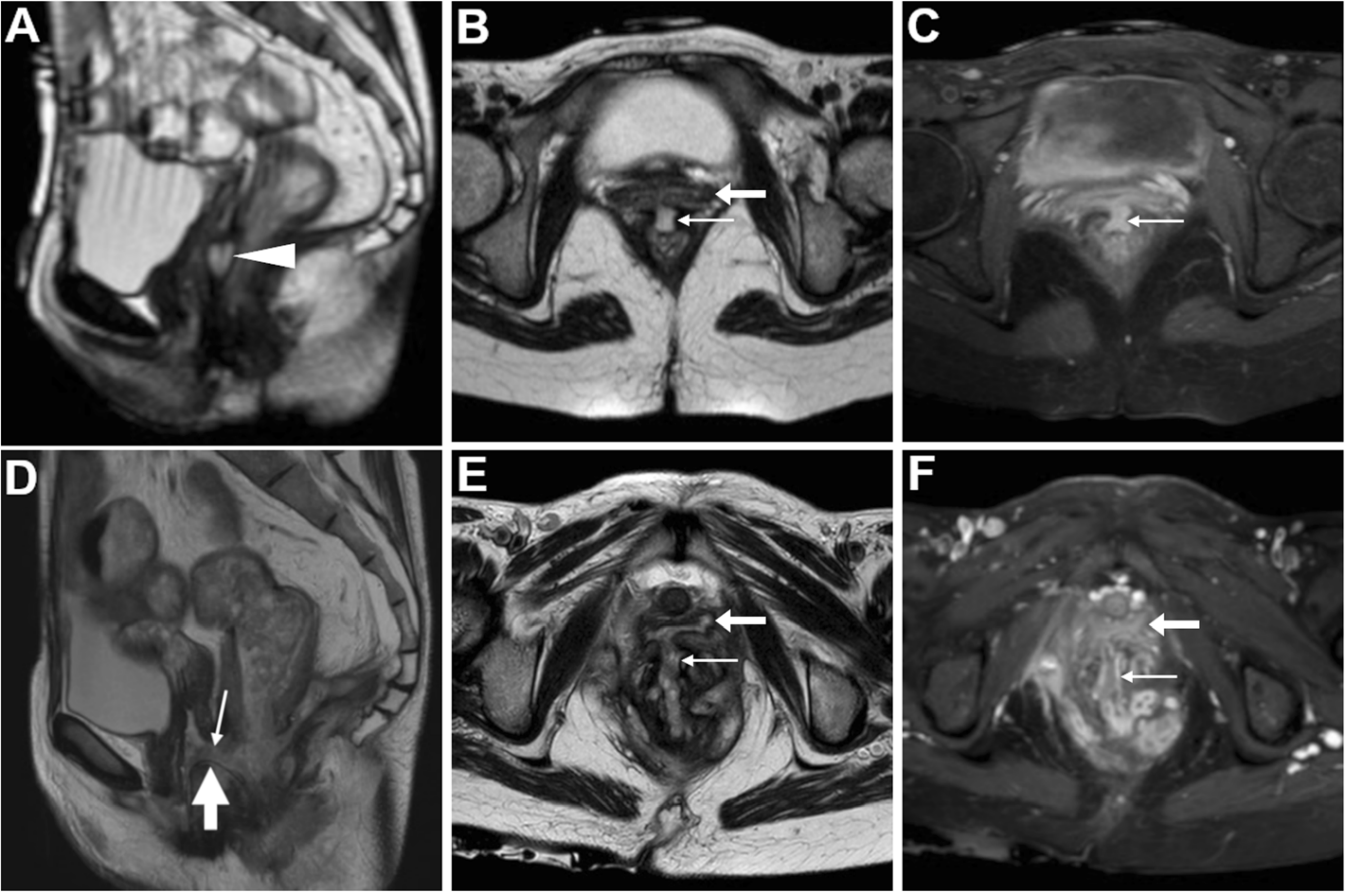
MRI follow-up of worsening perianal inflammatory disease in a 40-year-old with severe, refractory CD. Initial MRI (a-c) shows a small-sized abscess of the anovaginal septum (arrowhead in a) originating from a midline recto-VF (thin arrows) with strong enhancement on FS T1-weighted image after gadolinium CM (c). Two years later, despite positioning of a seton (thick arrow in d), repeated MRI (d-f) shows development of complex perianal disease including widened recto-VF (thin arrows) and formation of abscesses in both levator ani muscles. Note vagina (arrows)
